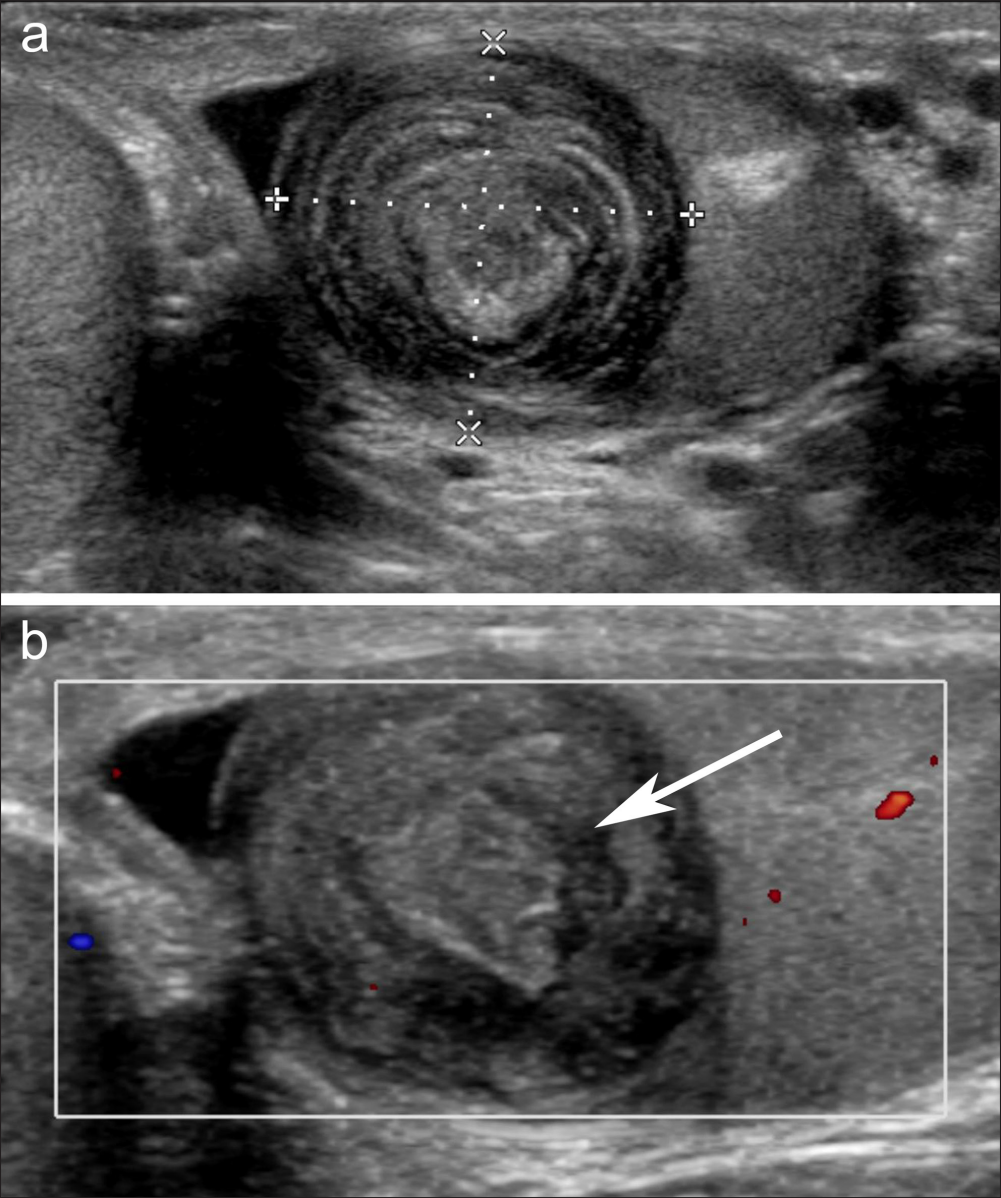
(a) B-mode sonography of the left testis shows an intratesticular mass with concentric rings of alternating echogenicity with a more echogenic central core. (b) Color Doppler sonography shows the absence of a vascular signal within the mass and a more echogenic central core (arrowhead).
